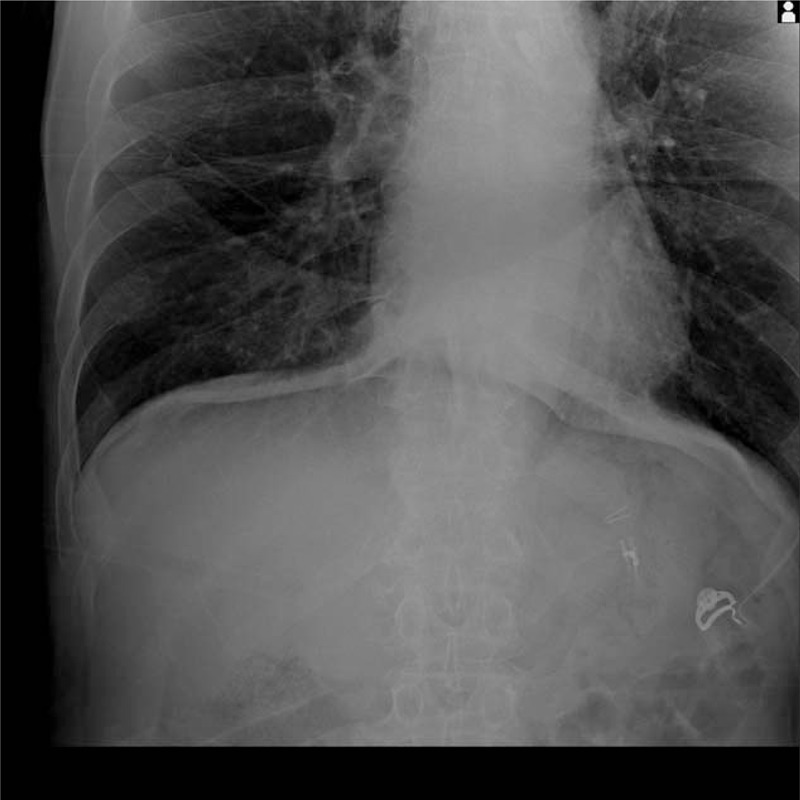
Intraoperative radiography showing the location of the metallic clips and endoscopic clips.
